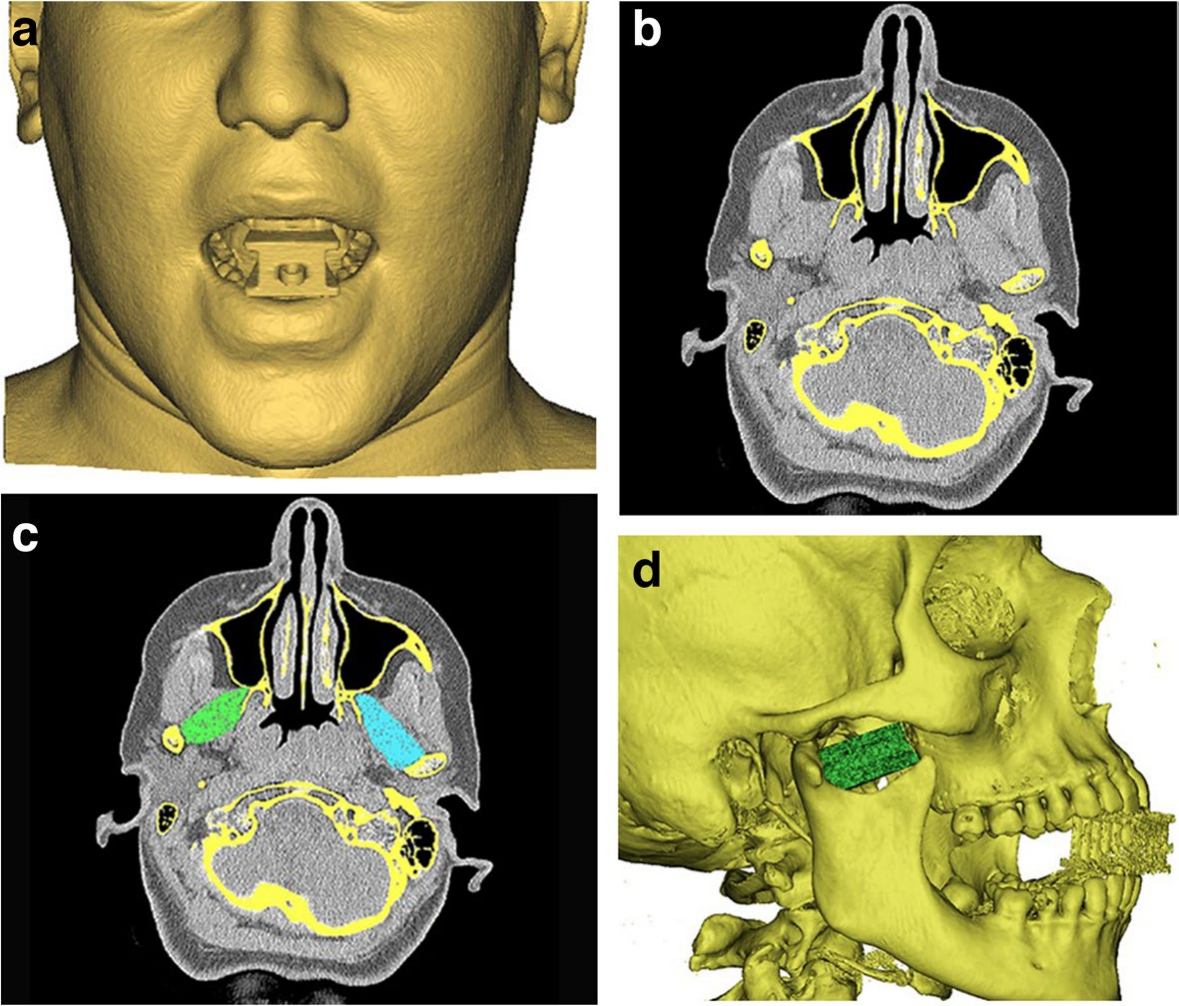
a: CT scan with soft tissue window on patient’s face while biting on a readymade bite block on the anterior teeth to prevent inter-arch teeth contact. b: Axial CT section showing right and left LPMs before segmentation. c: Right and left LPMs after segmentation in the same axial CT section d: 3D augmented model showing skull bone and teeth together with segmented LPM in its exact position
